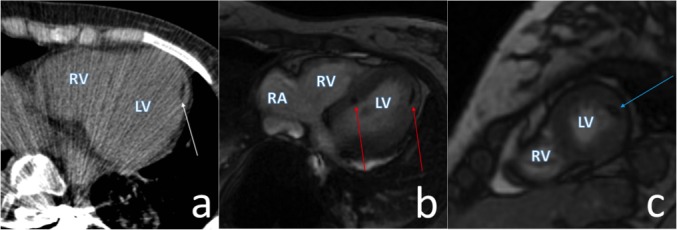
Cardiac CT and MR of Myocardial Fatty Foci (a) Axial noncontrast CT of the heart shows mid myocardial MFF deposit in the lateral wall (white arrow). (b) A bSSFP cardiac MR image in a four-chamber horizontal long axis view demonstrates myocardial focal fatty deposits with associated chemical shift artifact (red arrows). (c) Similar findings demonstrated on the two-chamber short axis view (blue arrow). bSSFP = balanced stready-state free precession; RA = right atrium; RV = right ventricle; LV = left ventricle.
